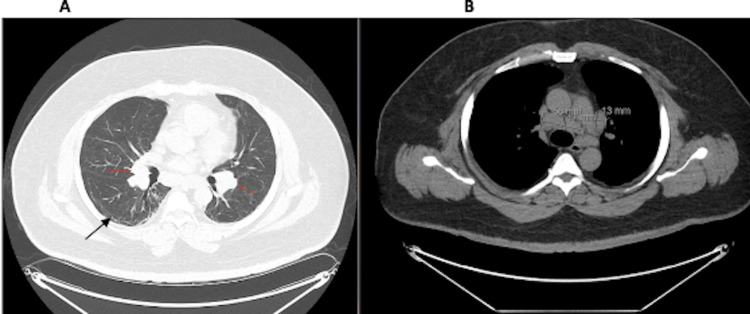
Computed tomography scan of the chest Pulmonary fibrosis (black arrow in image A) and mediastinal lymphadenopathy (red arrows in image A and measured lymph nodes in image B)
